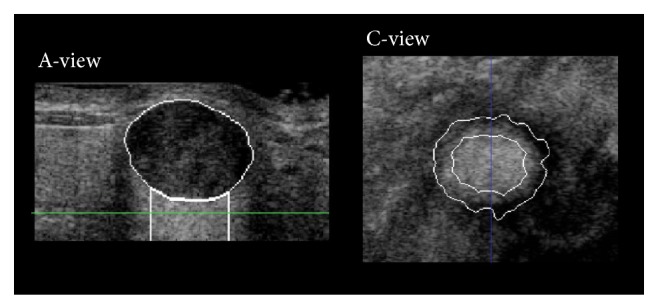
Sonographic A-view and C-view images of a tumor mass and its posterior region. The posterior region is the area under the tumor in the A-view image. The C-view image shows the section contour of the tumor lesion (external curve) and the section contour of the posterior regions (internal curve). The blue line in the C-view image indicates the plane of the A-view image. The green line in the A-view image indicates the plane of the C-view image.
